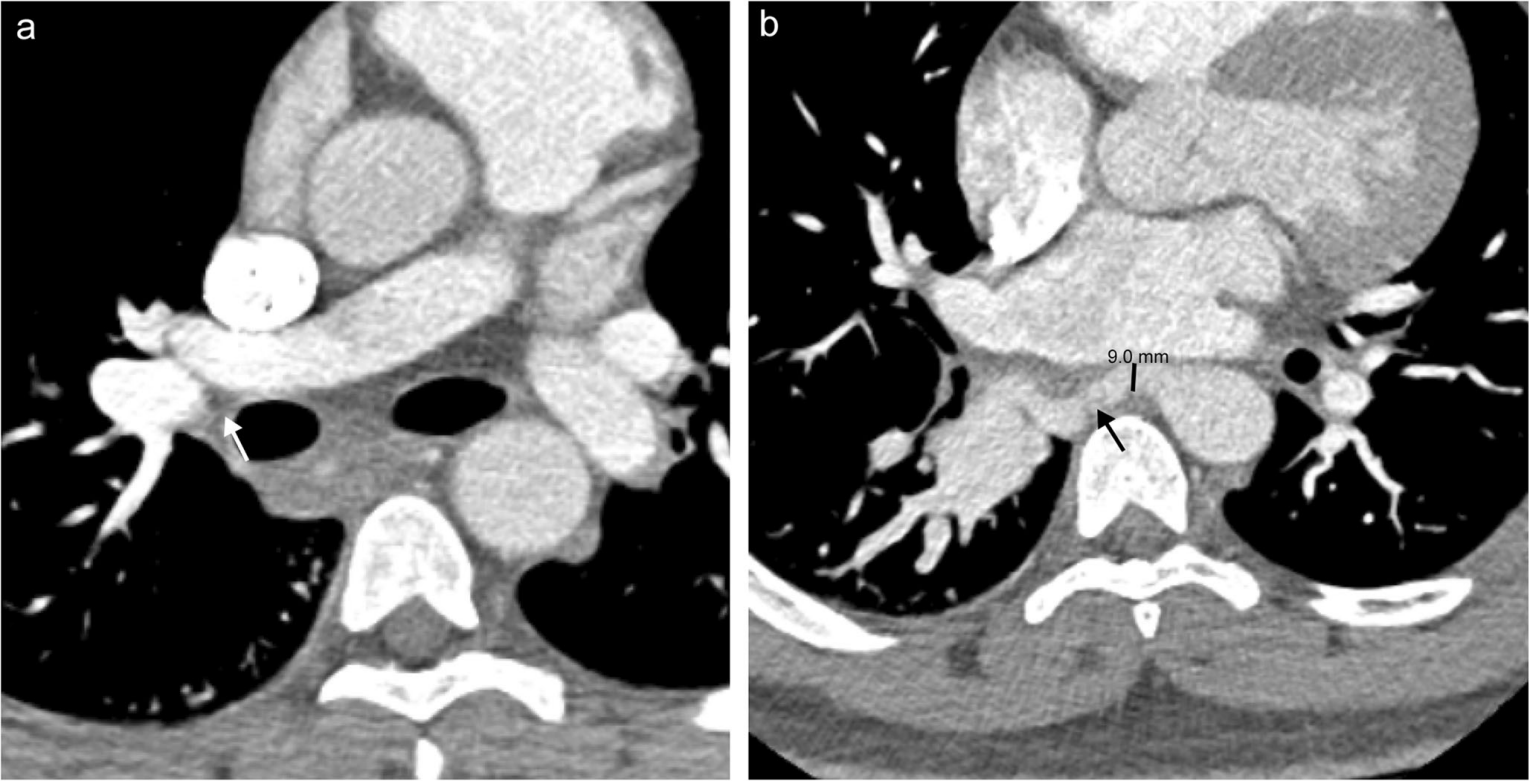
Bronchial artery dilatation. a Axial CT image showing congenital absence of the right lower lobe pulmonary artery (*white arrow*). b Axial CT image showing 9.0 mm dilated bronchial artery supplying the right lower lobe (*black arrow*)
